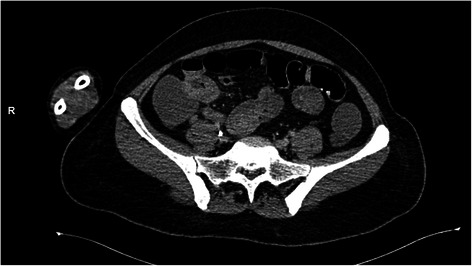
Abdominal computed tomography of the abdomen showing regional adenomegalies
